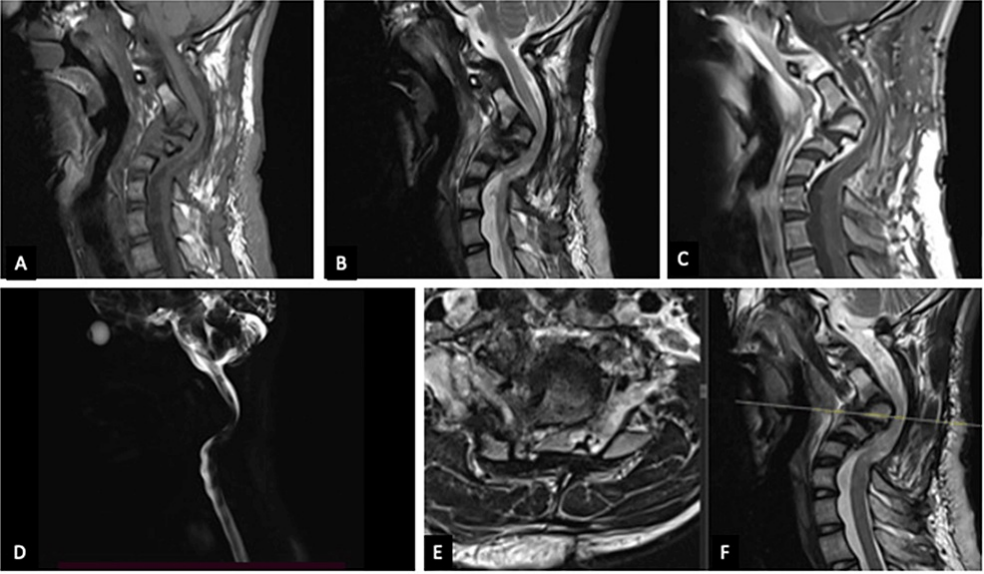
MRI of the cervical spine showing severe retrolisthesis of C3, severe kyphosis, complete cut-off in myelogram, cord compression, and myelomalacia. (A) T1-weighted image. (B) T2-weighted image. (C) T1 contrast image. (D) Myelogram. (E, F) T2-weighted axial image with corresponding sagittal cut.
